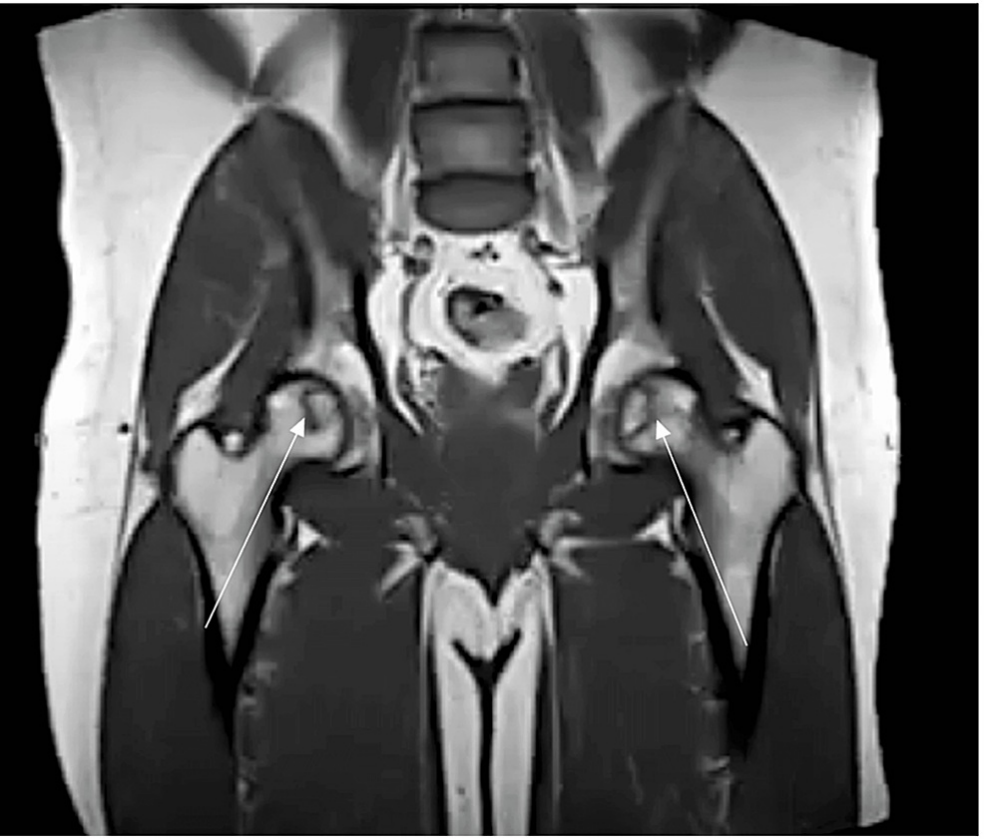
MRI of the anteroposterior pelvis. Arrows indicate stage II bilateral femoral head and left distal femur avascular necrosis without collapse on MRI.
